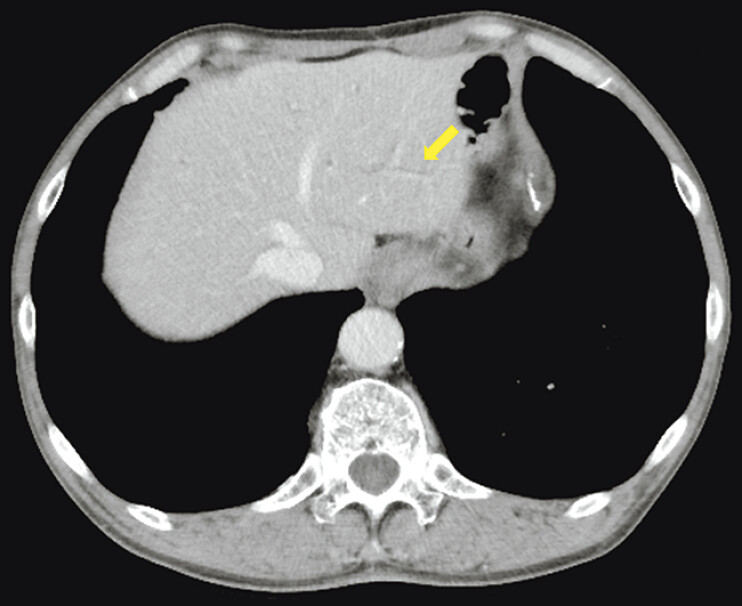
Abdominal computed tomography showing a minimally dilated B2 bile duct (arrow).
